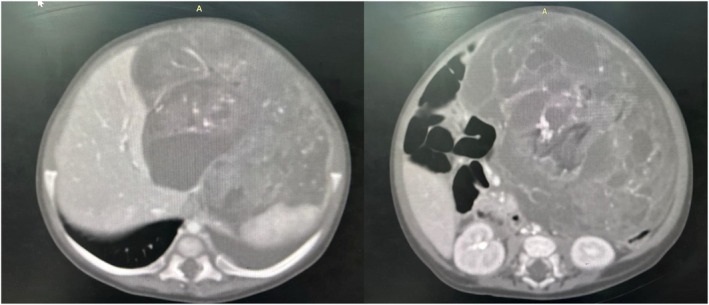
CT scan of the abdomen, showing a large, complex, heterogeneously enhancing lesion in the left hemiabdomen.
